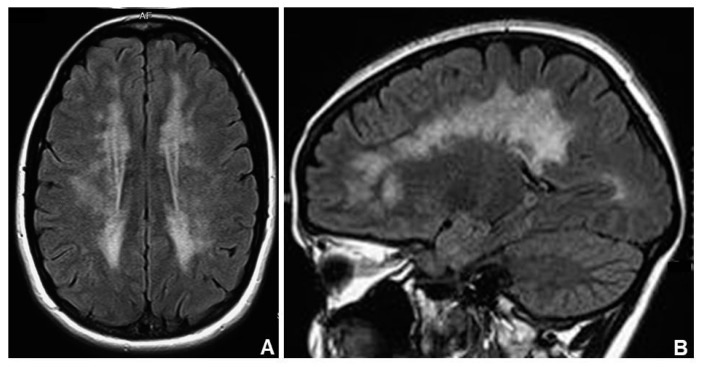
Axial (A) and sagittal (B) brain MRI (magnetic resonance imaging) view shows marked leukoencephalopathy in a MNGIE (Mitochondrial Neurogastrointestinal Encephalomyopathy) subject.
